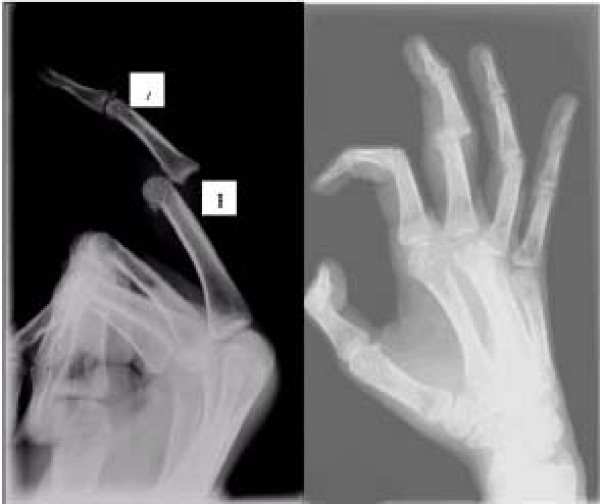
X-ray of lateral and oblique view. Note the avulsed bony insertion of extensor of the distal phalanx. (A). volar plate fracture (B).
